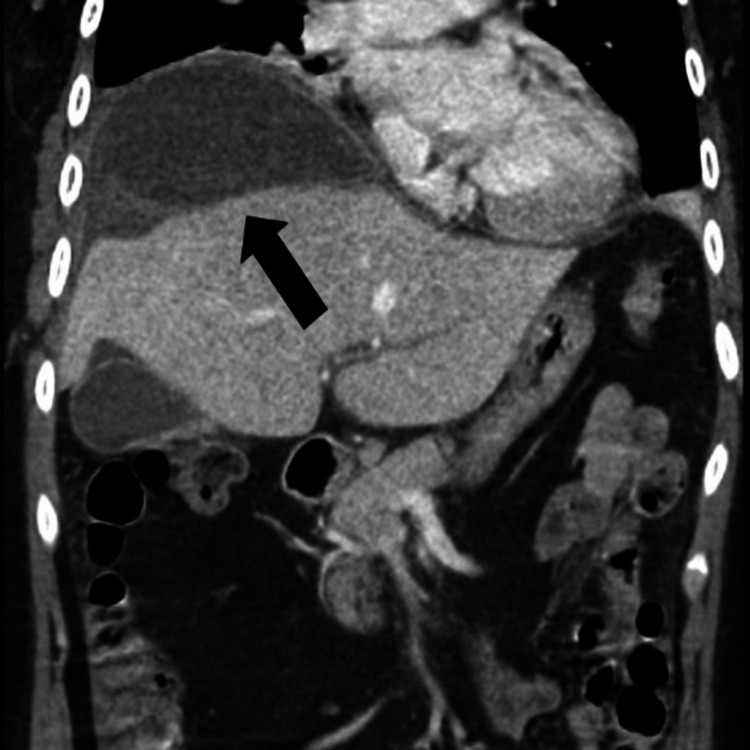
CT abdomen and pelvis, coronal image, shows a new peri-hepatic collection surrounding the hepatic dome (arrow).
